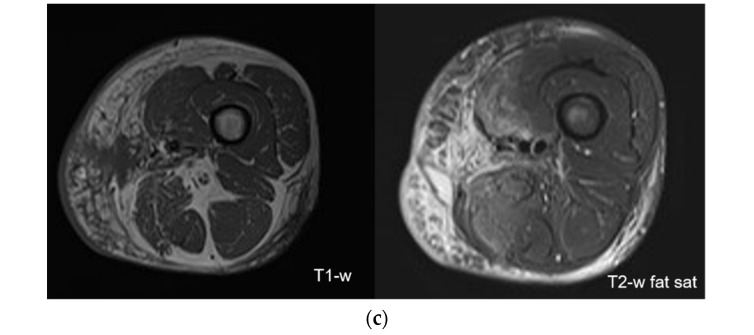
A 45-year-old man operated for a large MPNST with translocation t(X; 18) (p 11.2; q 11.2) and of genes SYT/SSX of the popliteal fossa shown by both US (a) and MRI (b). Follow-up did not show any changes consistent with relapse; only minor edema and fluid collection were present (c). Both MRI and US were reported negative for recurrence.
